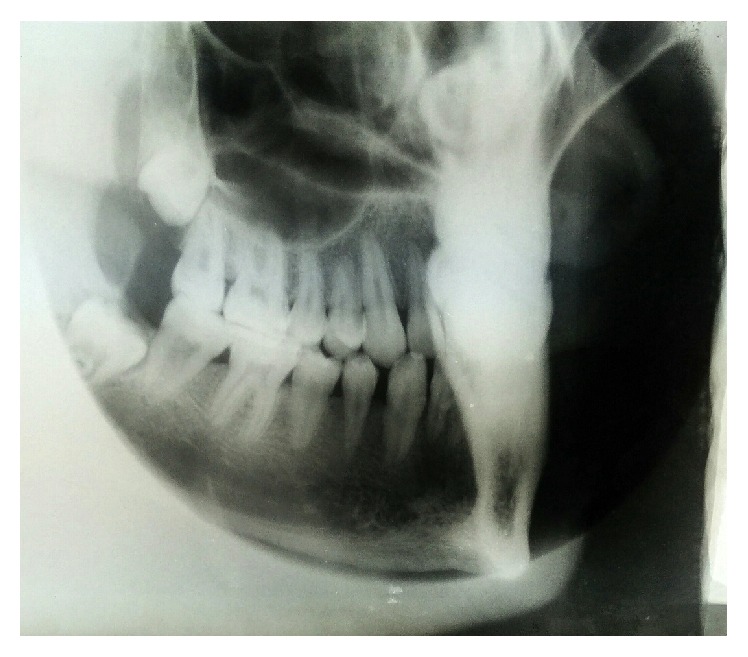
Preoperative lateral radiography of right side mandibular wisdom tooth.
